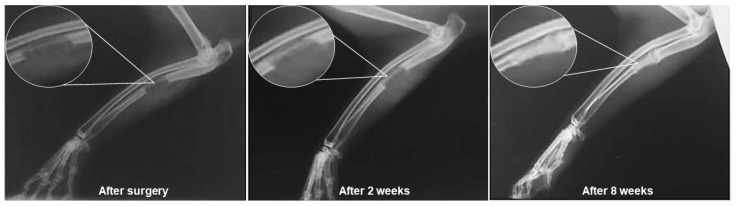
Radiographs of the ulnar segment defect immediately after surgery, after 2 weeks and 8 weeks. Adapted from Hafezi et al. [198].
